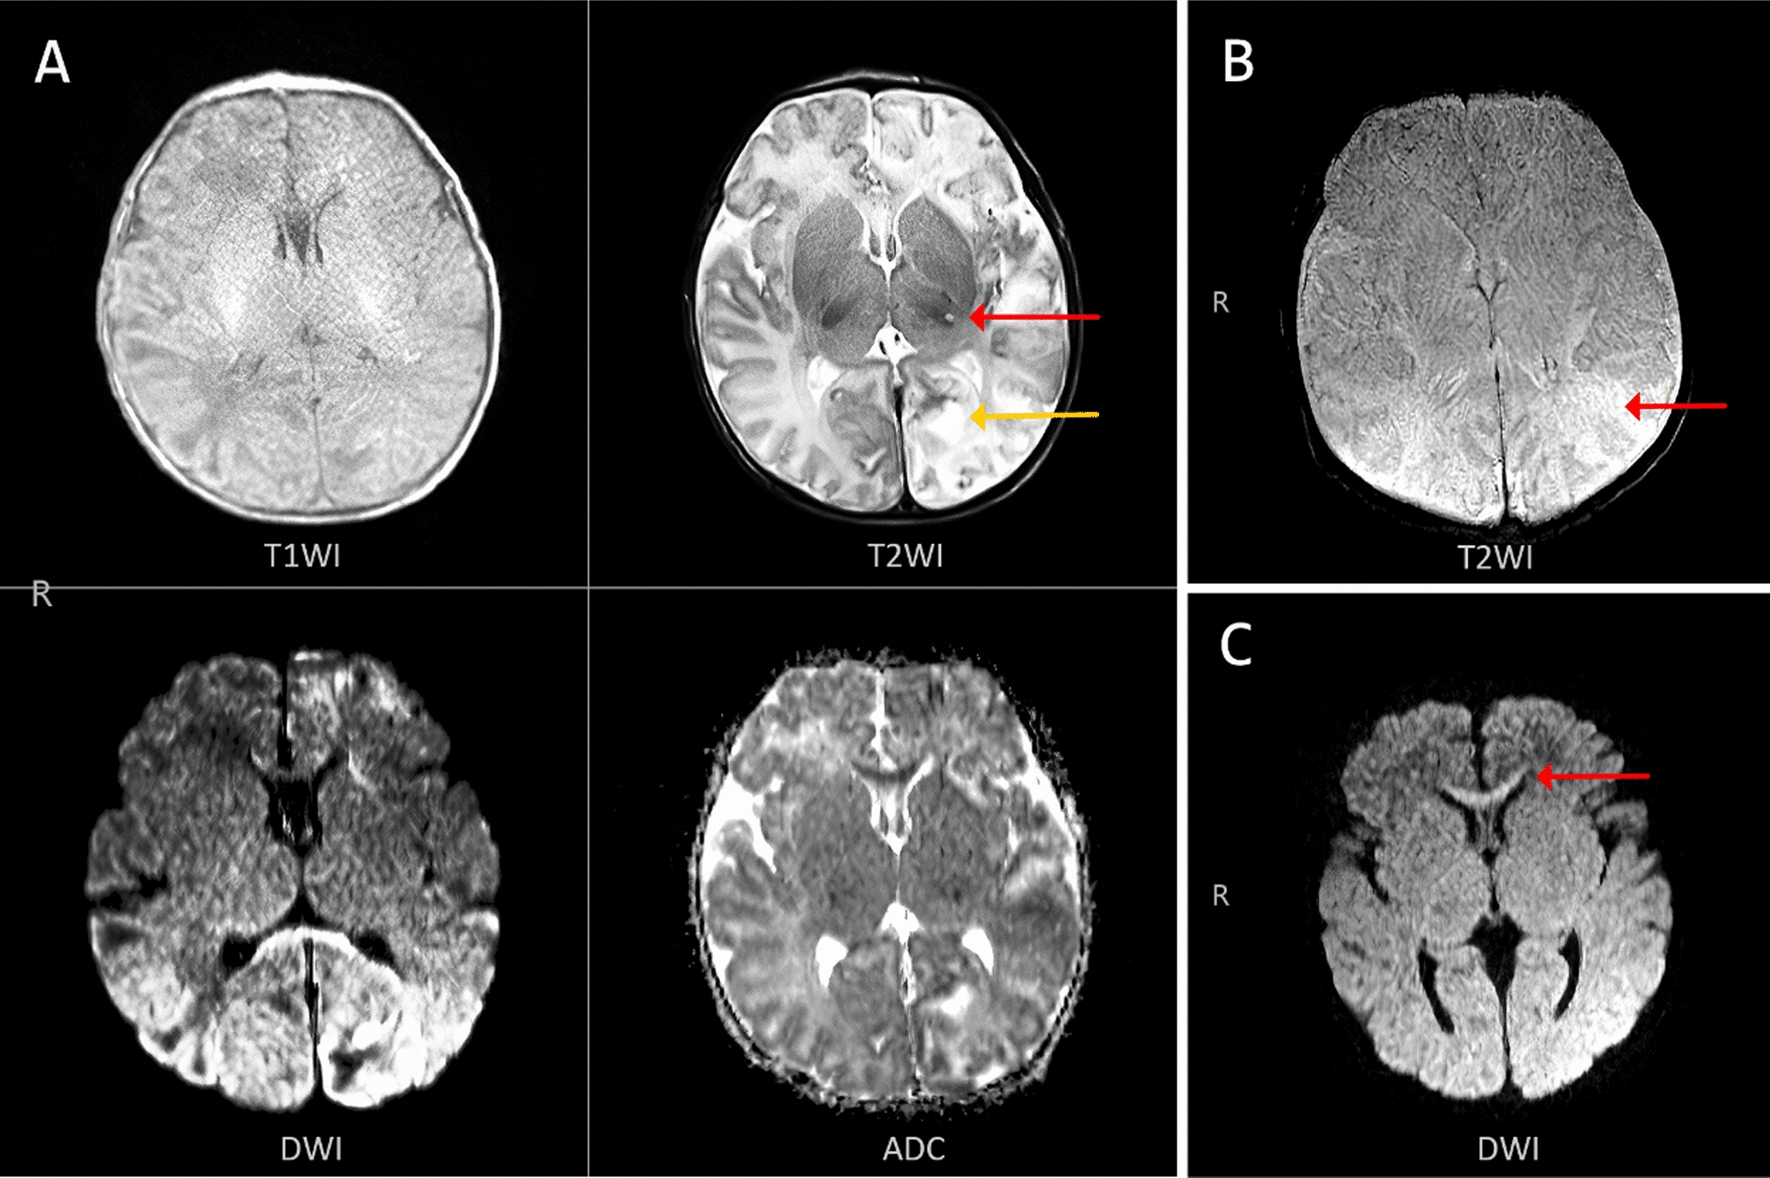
MRI of brain ischemia and hemorrhage: A The MRI of the 14th patient showed T1- and T2-weighted extensive signal intensity changes. Diffusion Weighted Imaging(DWI) and Apparent Diffusion Coefficient(ADC) showed high-signal-intensity lesions in the left cerebral hemisphere and right frontal lobe, which were proposed to be hypoxic infarction. B The MRI of the 31th patient showed occipital subdural hemorrhage. C The MRI of the 14th patient showed genu of the corpus callosum depicting high signal intensity
